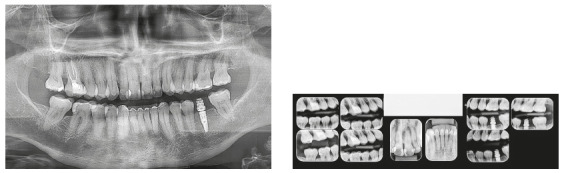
Final radiographs of Case 4.
